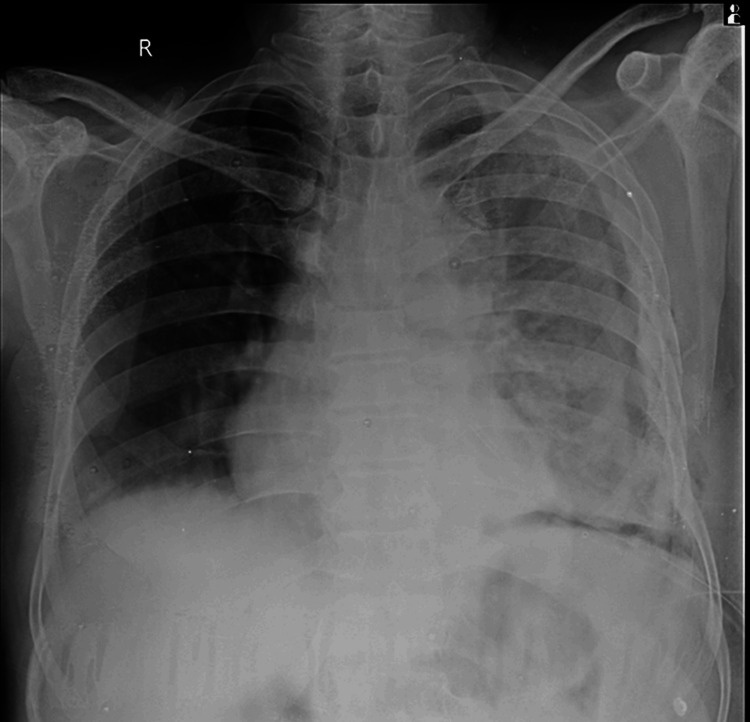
Chest X-ray on discharge post-operative day 10 showing good lung expansion on the left side
